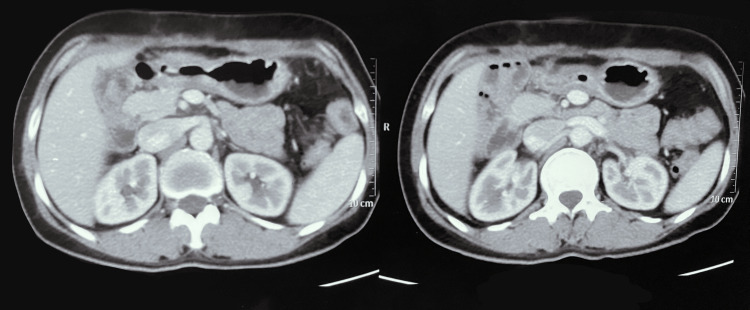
Follow-up CECT abdomen and pelvis shows no evidence of recurrence CECT: Contrast-Enhanced Computed Tomography
